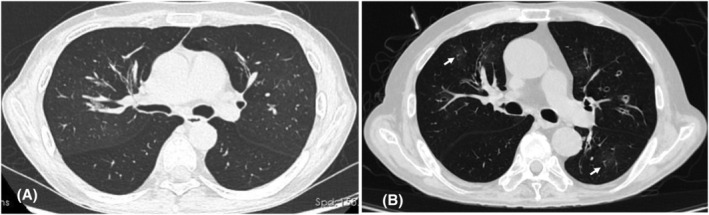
(A) Case 1 high‐resolution computed tomography (HRCT) showed mild, diffuse bronchial wall thickening. (B) Case 2 HRCT presented mild, diffuse bronchial wall thickening with mild bronchiolectasis and patchy centrilobular ground‐glass opacities (arrow)
